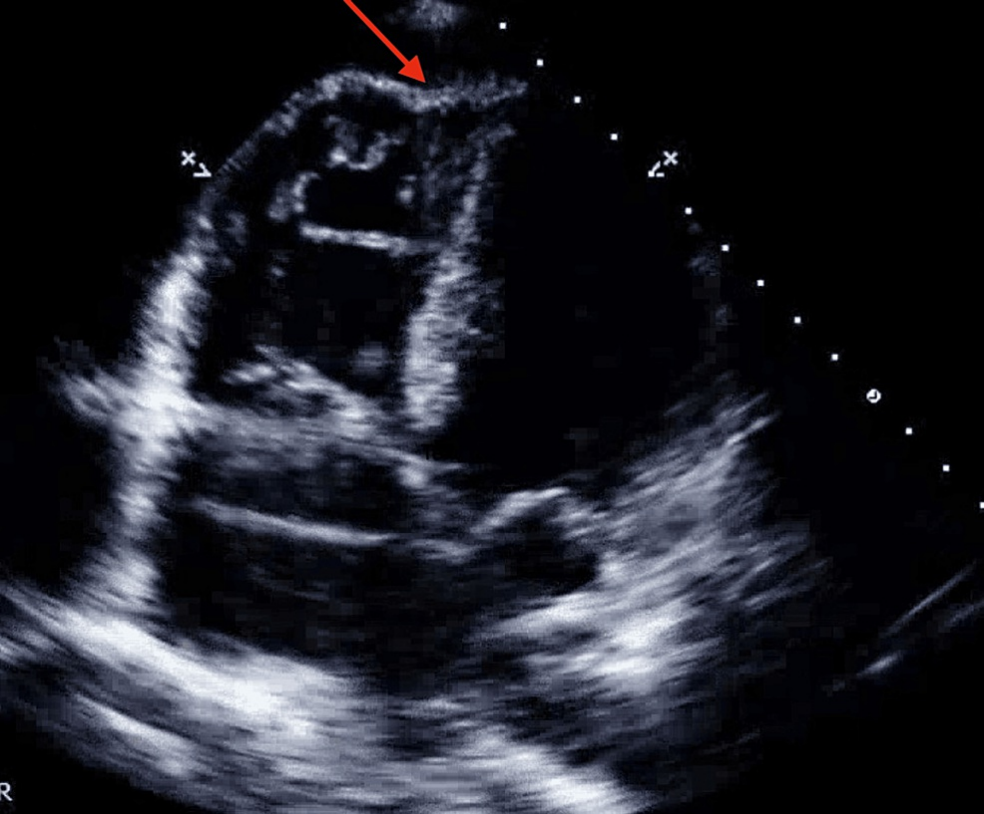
A bedside TTE revealed a possible McConnell sign, as shown by the red arrow. TTE: transthoracic echocardiogram
